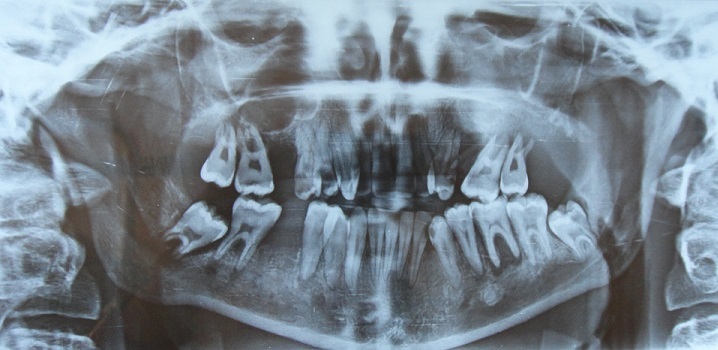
Radiographie panoramique montrant des lésions ostéolytiques avec disparition de la lamina dura et élargissement desmodontal au niveau des molaires supérieures et inférieures
